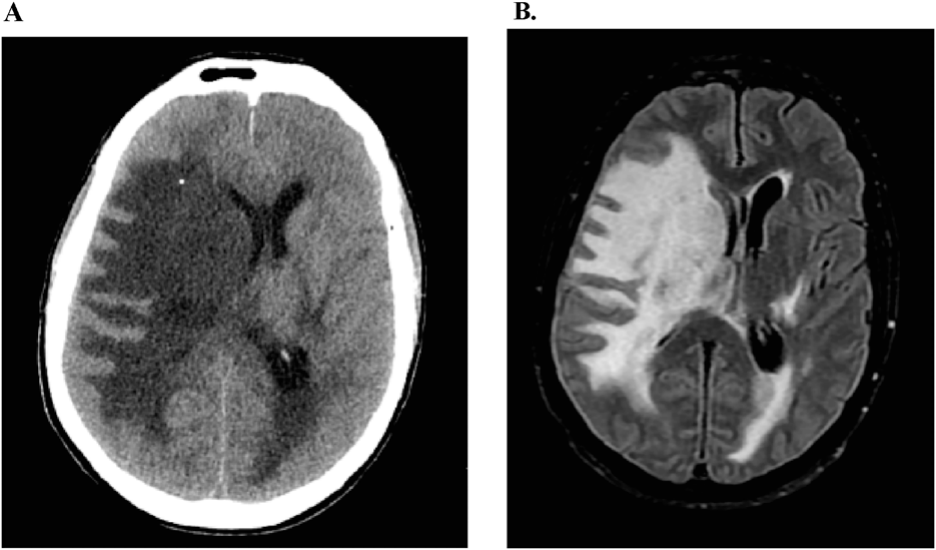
(A) Head CT at the time of presentation showing significant vasogenic edema burden at the subcortical white matter with associated 11 millimeter right to left midline shift at the level of the lateral ventricles. (B) MRI brain without contrast in T2 FLAIR sequence on day 2 of hospitalization after receiving 1 dose of methylprednisolone. Imaging was notable for extensive vasogenic edema bilaterally with the right side worse than left. There was an 8 millimeter right to left midline shift present and mild dilation of the left lateral ventricle concerning for entrapment.
